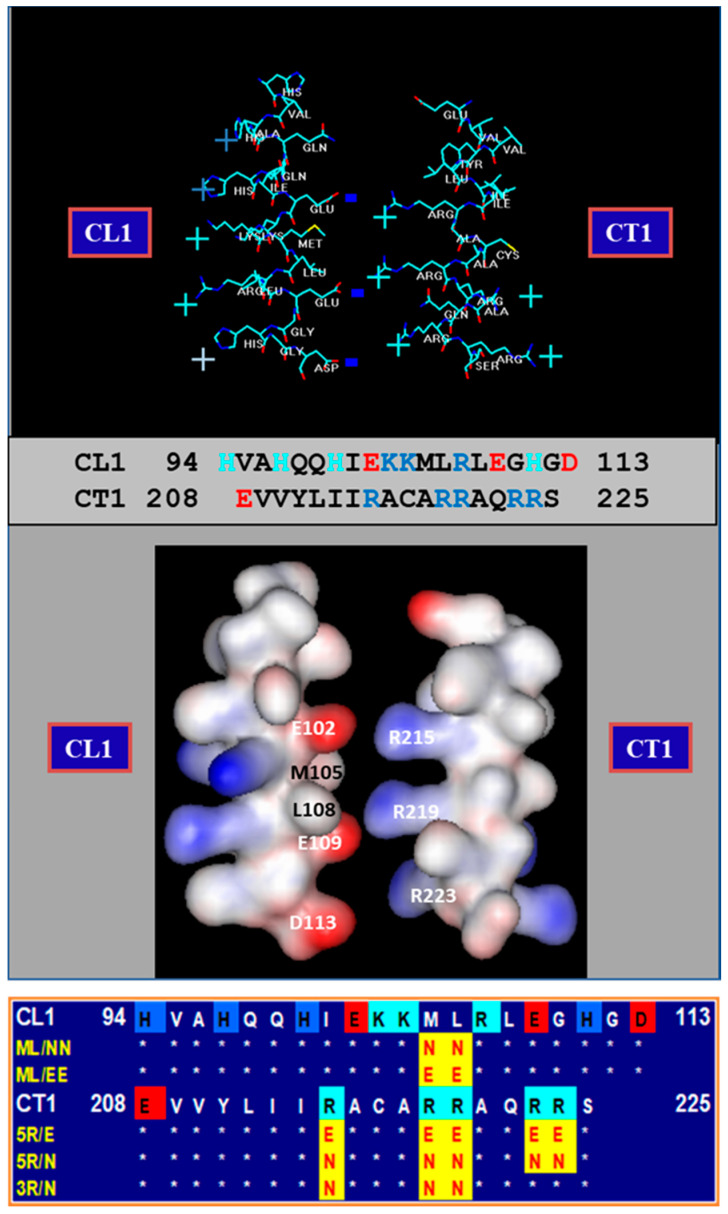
Cx32′s CL1 (first half of CL) and CT1 (initial domain of CT) domains in α-helical conformation. Our hypothesis is that in coupled conditions CL1 and CT1 interact electrostatically (negative CL1, positive CT1) and hydrophobically. CL1 and CT1 mutation (bottom panel) are believed to prevent the interaction allowing the negatively charged CaM’s N-lobe to access the positively charged channel’s mouth and plugging it by interaction with it electrostatically (CaM-Cork model). We suggest that with an increase in [Ca^2+^]_i_ the activated CaM’s N-lobe accesses the gating site and plugs the channel’s mouth by breaking the CL1-CT1 interaction (Ca-CaM-Cork model).
